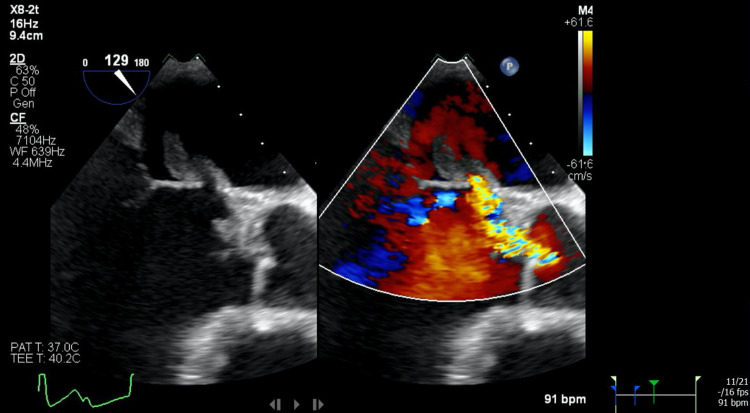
(Left) Transesophageal echocardiogram image showing large vegetation on the aortic and mitral valve; (right) transesophageal echocardiogram with color doppler showing severe aortic regurgitation
